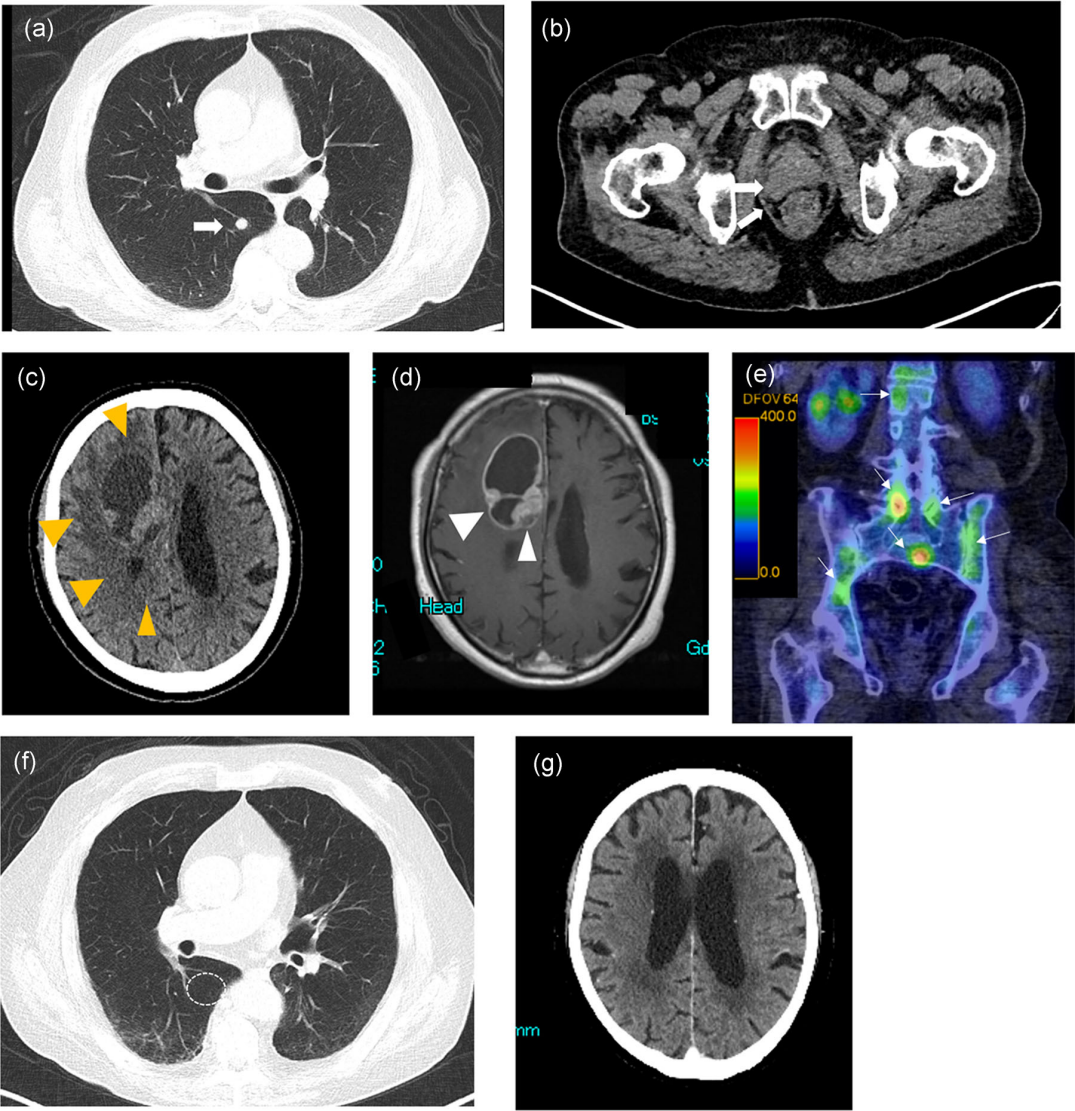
Diagnostic imaging findings on presentation. (a) PET image showing metastatic lung nodules in the right lobe. (b) CT image of the prostate showing the irregular shape. (c,d) Brain CT (c) and MRI (d) images showing signs of frontal lobe metastasis. (e) 99mTc‐HMDP bone scintigraphy revealing multiple metastases in the spinal bone. (e,f) Imaging results after treatment. Chest (e) and brain (f) CT after platinum‐based doublet chemotherapy combined with etoposide. CT shows partial remission of brain metastasis and complete remission of pulmonary metastasis. CT, computed tomography; MRI, magnetic resonance imaging; PET, positron emission tomography.
